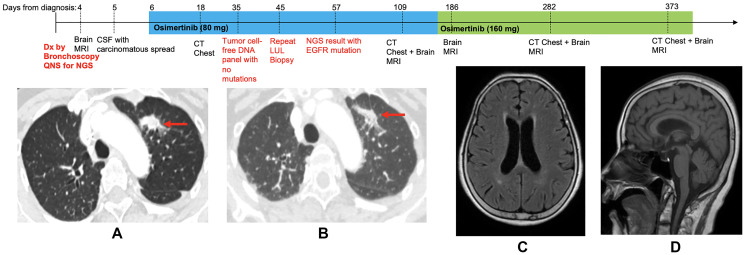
Treatment timeline with chest computed tomography (CT) and brain magnetic resonance images (MRI) on osimertinib. (A) Pretreatment CT chest, demonstrating 18 mm spiculated mass in the left upper lobe (LUL). (B) CT chest following 12 days of Osimertinib treatment, showing decreased size of LUL mass. (C) Pretreatment brain MRI showing no acute abnormalities (axial). (D) Pretreatment brain MRI showing no acute abnormalities (sagittal).
